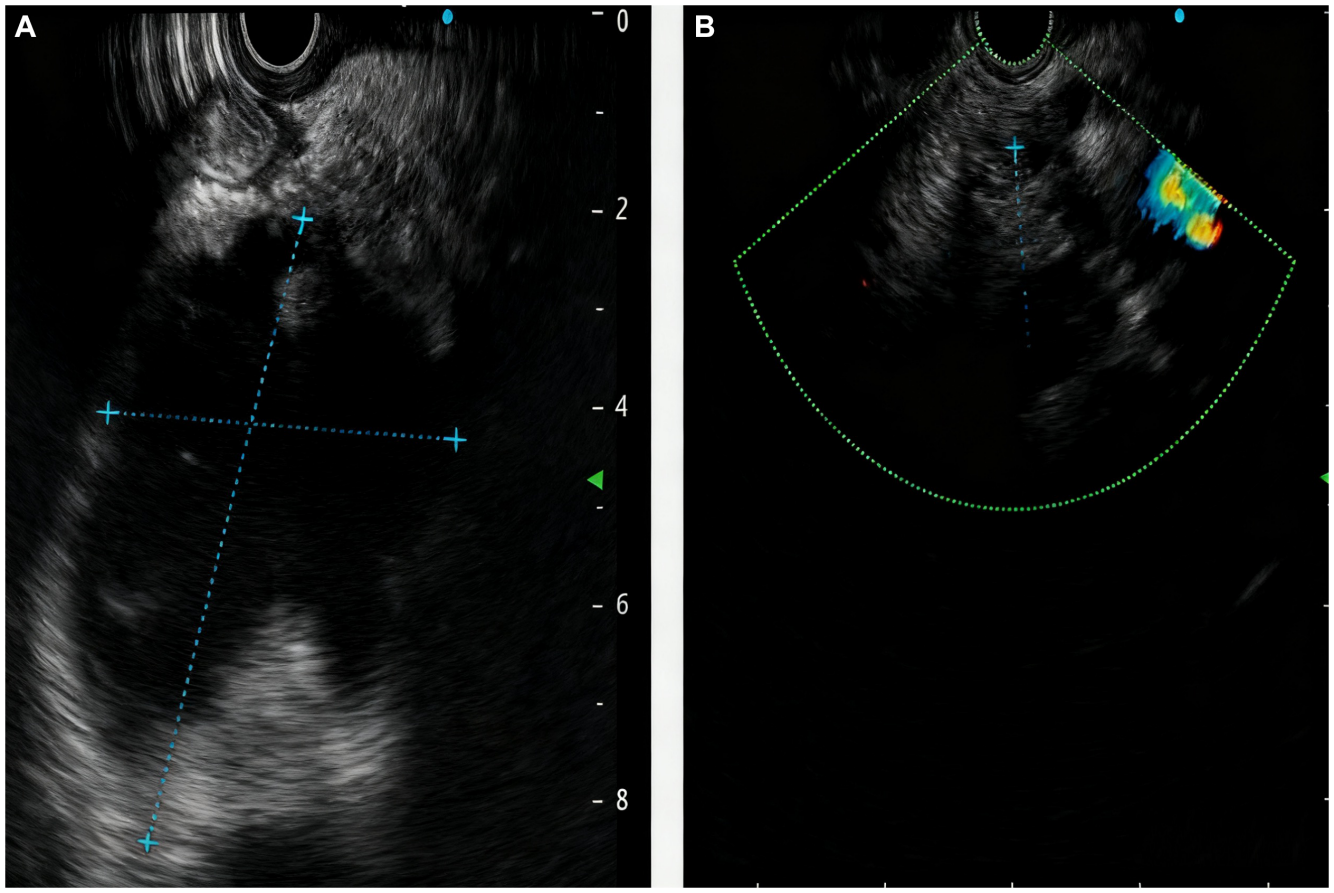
The manifestations of the pancreas under endoscopic ultrasound revealed the (A) a cystic lesion measured approximately 6.7cm x 5.4cm in its largest cross-sectional dimension, communicated with the MPD; (B) reduction in the size of pancreatic cystic lesion after 2 months.
